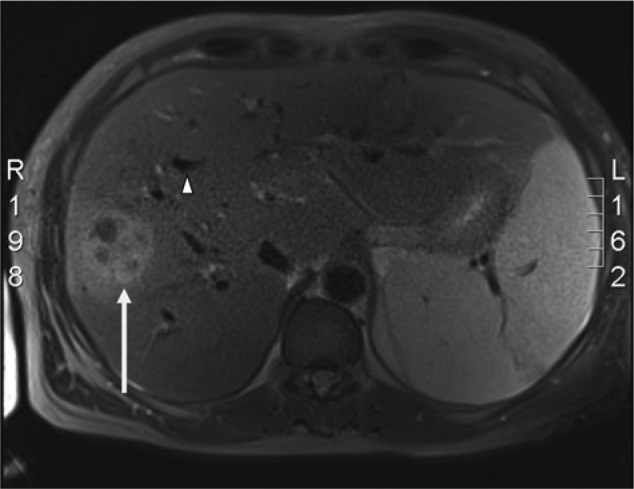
Liver magnetic resonance cholangiopancreatography. The space-occupying lesion seen on ultrasound (white arrow), consistent with a pyogenic liver abscess, can be seen in the right lobe of the liver; multiple dilated intrahepatic biliary ducts (white arrow head) are also present.
